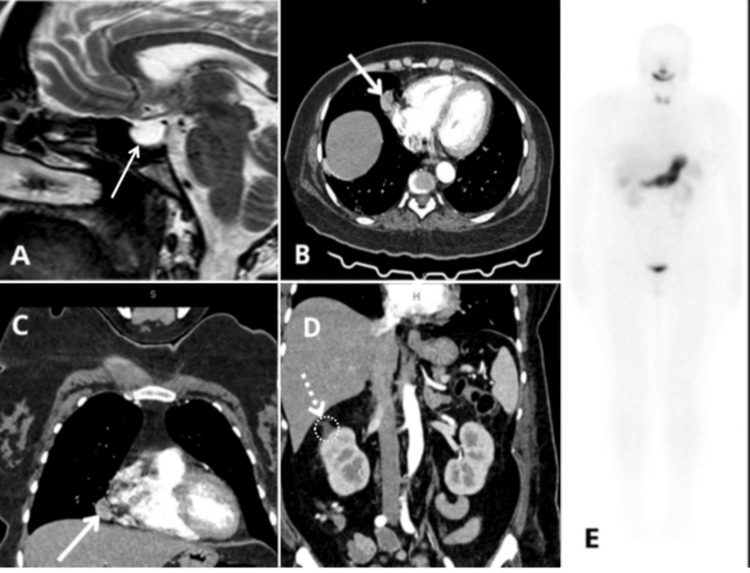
Serial CT and MRI imaging demonstrating multifocal lesions involving the pituitary gland, mediastinum, and adrenal gland (A) Sagittal T1-weighted brain MRI showing an expansive sellar arachnoidocele with downward displacement of the pituitary gland. (B, C) Contrast-enhanced chest CT in axial (B) and coronal (C) planes demonstrating an anterior mediastinal lymph node (arrows). (D) Contrast-enhanced abdominal CT revealing bilateral adrenal gland hyperplasia (arrow). (E) Somatostatin receptor scintigraphy with technetium-99m (^99m^Tc)-labeled octreotide showing no evidence of residual or metastatic disease.
